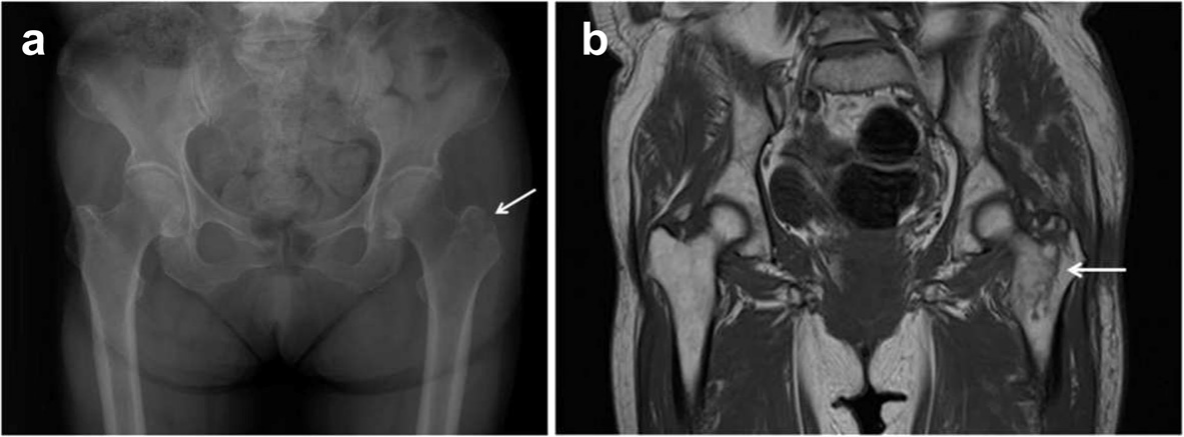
a AP radiograph of a 75-year-old woman shows only a minimally displaced isolated fracture of the GT of the Lt hip. b MRI reveals a fracture from the GT leading toward the lesser trochanter (more than half way to the medial cortex)
